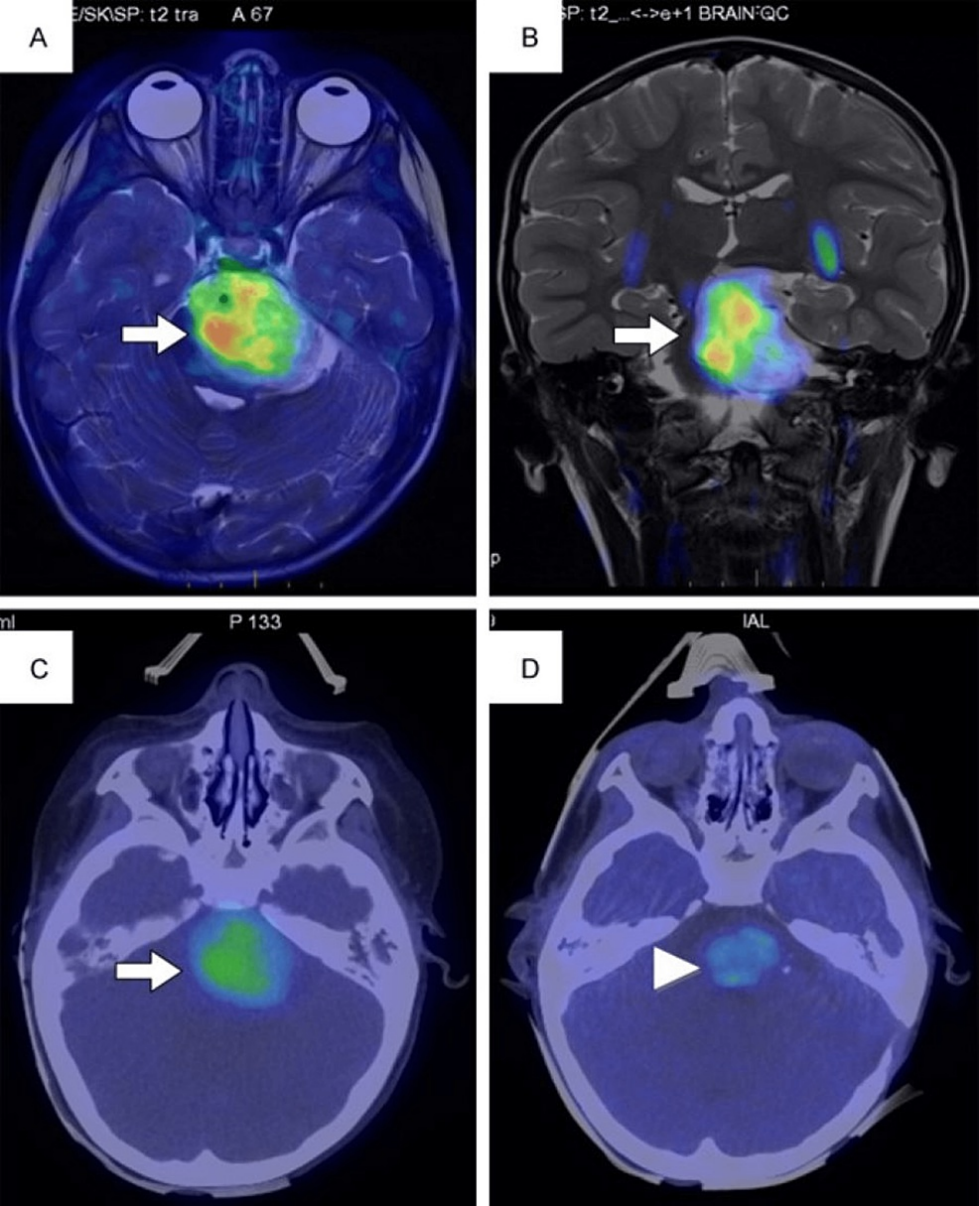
18F-DOPA scan of neuroendocrine embryonal tumor before and after therapy documenting treatment response ^18^F-DOPA: Fluorine-18-I-Dihyroxyphenylalanine Axial (A) and coronal (B) magnetic resonance imaging - positron emission tomography fusion images and axial positron emission tomography/ computed tomography (C) images showing brainstem uptake (arrow); axial positron emission tomography/computed tomography (D) after systemic chemotherapy and proton therapy shows lower brainstem uptake (arrowhead) This scan image is reproduced from Masselli et al. [62] - permission obtained from e-Century Publishing Corporation
